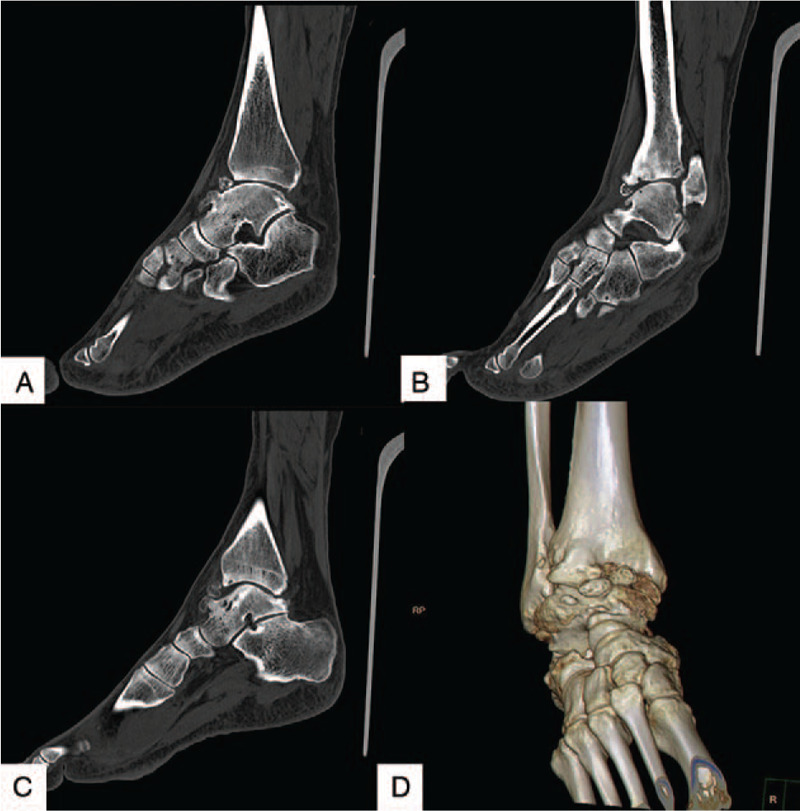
CT scan (A to C) showed sclerotic borders under cartilage and cystic changes in tibia and talus. It also revealed narrowing joint space and osteophytes on the talus and around the ankle joint (D).
